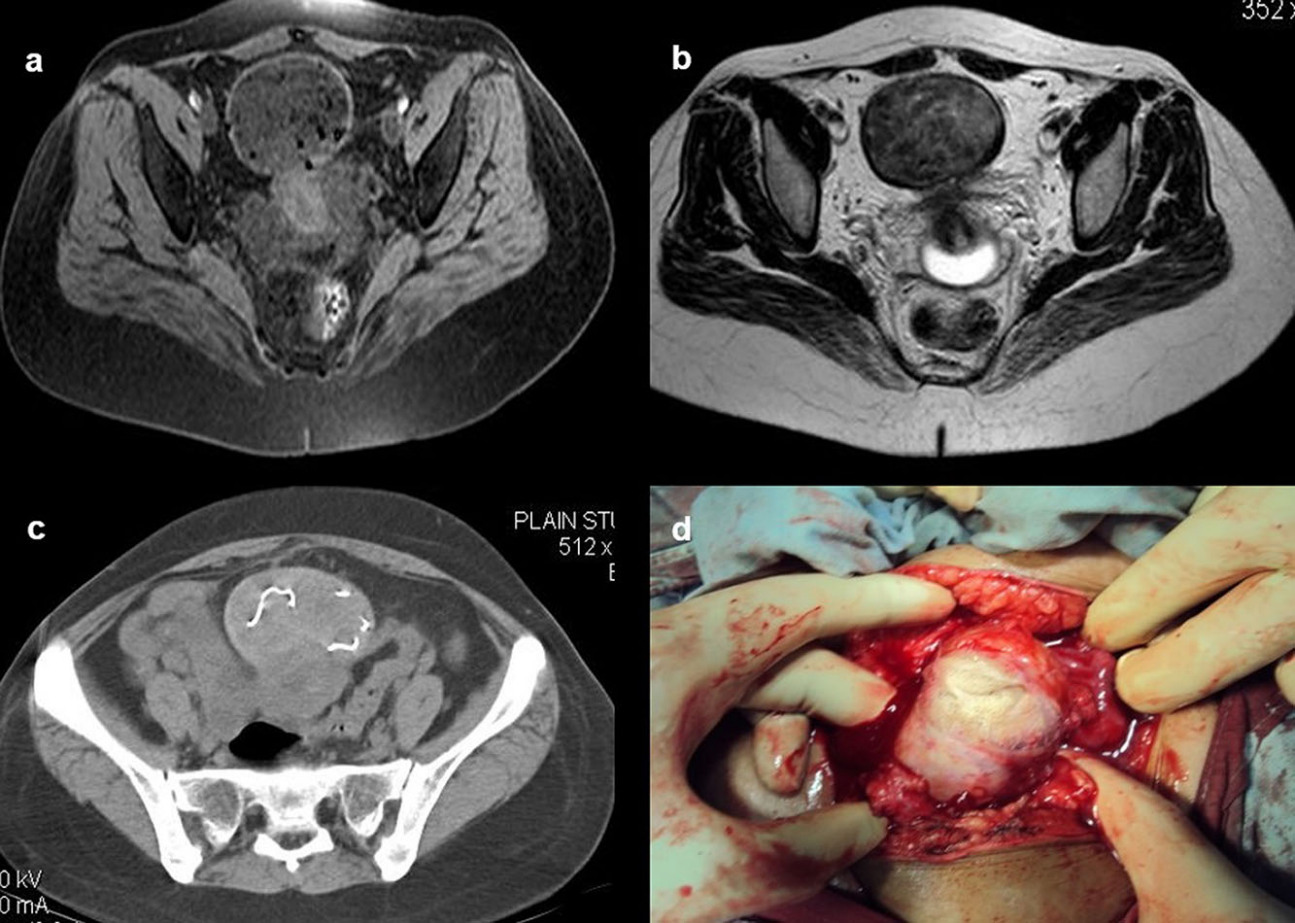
a-d: MRI axial T2 and T1 Fat Sat images shows heterogeneous altered signal intensity soft tissue mass anterior to the uterus with multiple hypointense foci within (a, b) CT showed multiple linear metallic strings within a soft tissue density mass anterior to the uterus-gossypiboma (c). The surgical sponge was removed at surgery (d).
